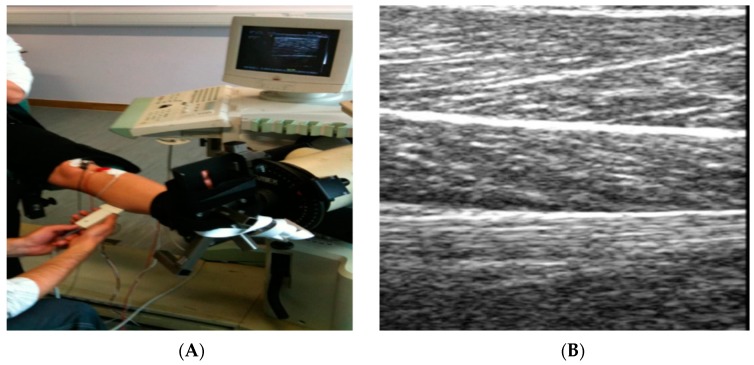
(A) Application of ultrasound and equipment involved; (B) Example of a grey scale image produced by an Ultrasound when placed directly upon skeletal muscle.
